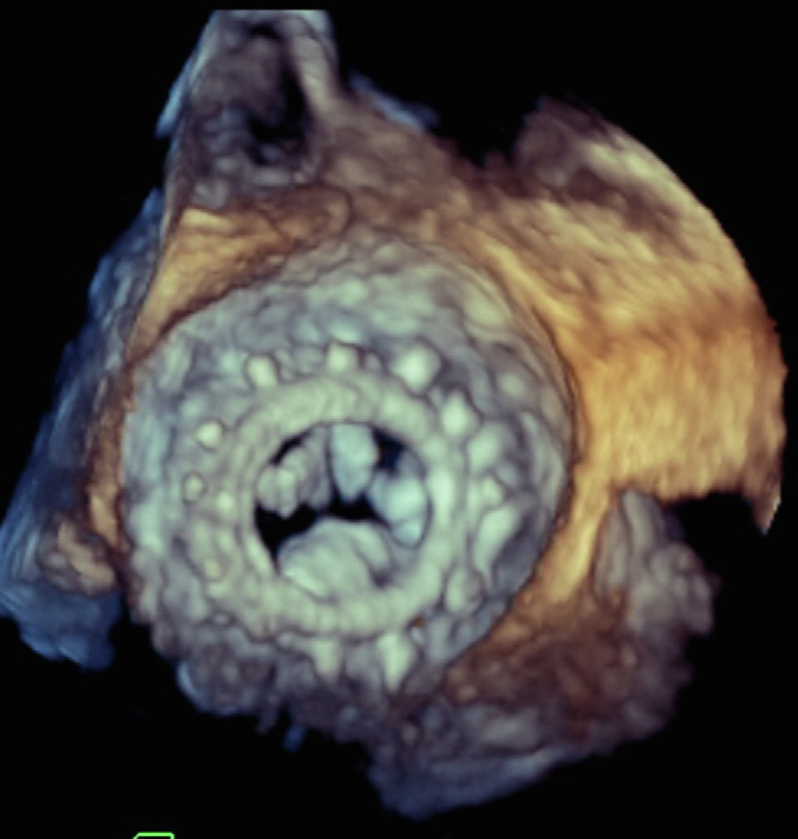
Prosthetic Mitral Stenosis A 3-dimensional transesophageal echocardiogram demonstrating thickened prosthetic mitral valve leaflets. The mitral valve area by planimetry was measured to be 1 cm^2^.
